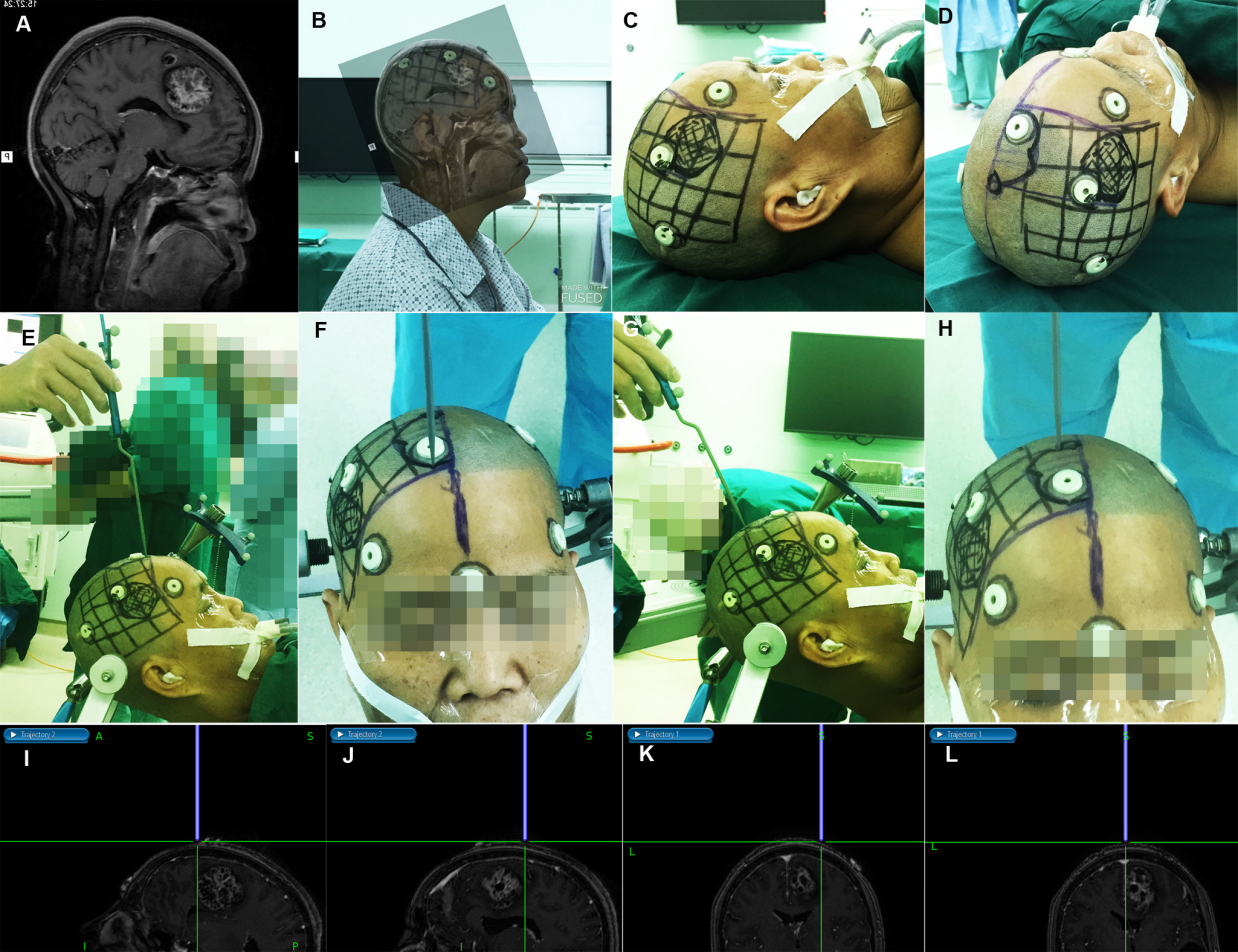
Comparing the new method with a frameless neuronavigation system. (A) Mid-sagittal T1 image of patient 6 in the second-stage trial, showing a cavernous angioma in the frontal lobe. (B) Co-registration of the mid-sagittal MR image with the sagittal photograph of the patient. (C) The lesion’s sagittal projection was marked on the skin according to the co-registration results. (D) In accord with the sagittal projection of the lesion, the axial projection was also depicted on the skin. (E) and (F) After anesthesia and co-registration with the navigation system, the operator pointed the navigation probe to the anterior boundary of the lesion. The probe direction was adjusted to be parallel with the sagittal plane and vertical to the mid-sagittal line. (G) and (H) The operator pointed the navigation probe to the posterior boundary of the lesion. (I) On the display screen, the “trajectory 2” navigation mode was chosen; The thick blue line indicated the navigation probe, which was vertical to the head surface. Its extended line, which touched the anterior boundary of the lesion, was green. The deviation was 0 mm. (J) The deviation at the lesion’s posterior boundary was also zero. (K) The “trajectory 1” model was chosen, and the deviation at the lesion’s lateral boundary was 2.3 mm. (L) The deviation at the lesion’s medial boundary was 0 mm.
